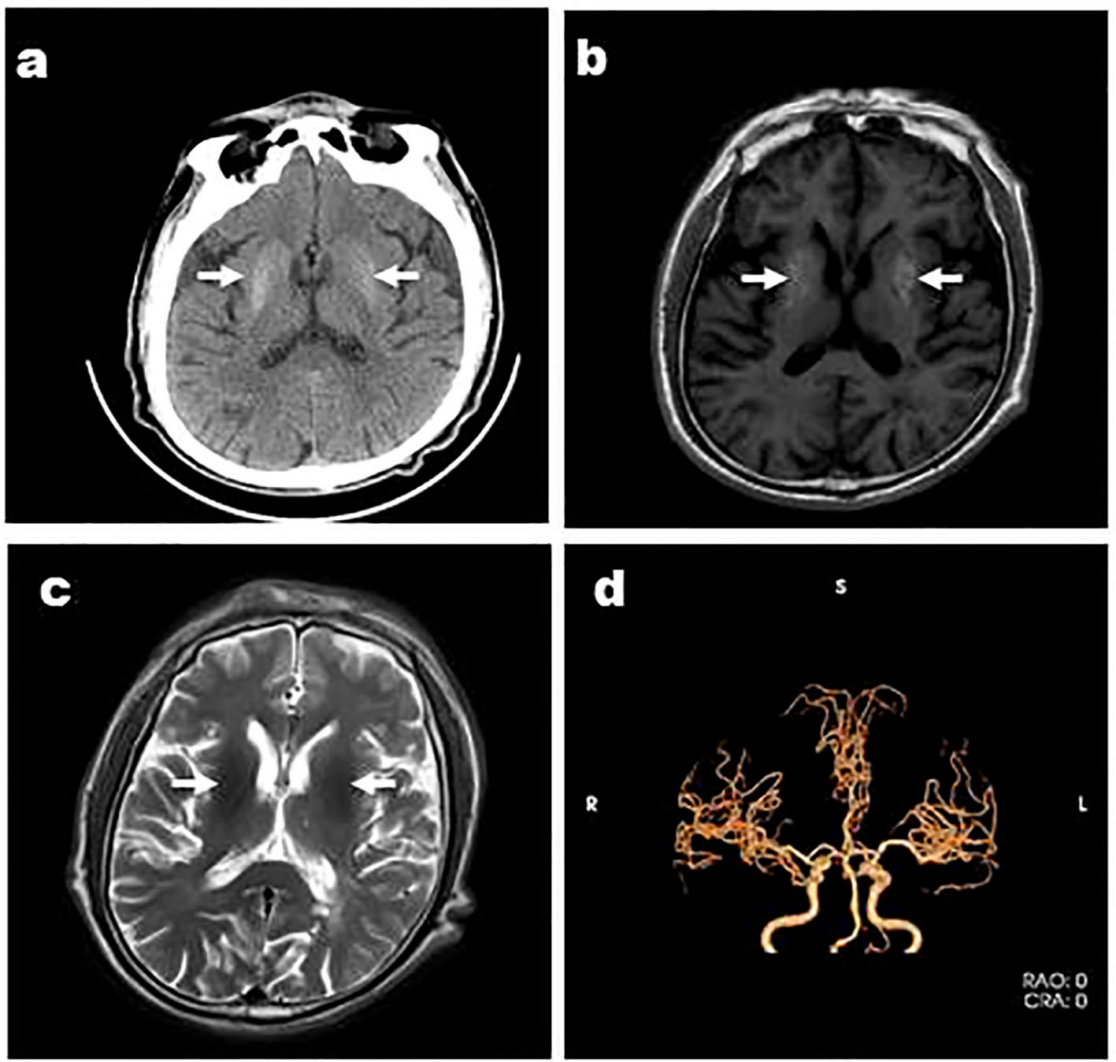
Case 3, a 66-year-old male, was admitted with “involuntary movement of the tongue and right limb for more than 1 month” and images showed bilateral striatum high density on CT (A), high signal on T1WI (B), iso-signal on T2WI (C), and MRA showed arterial stenosis (D). Arrows indicate bilateral striatum abnormal signal.
